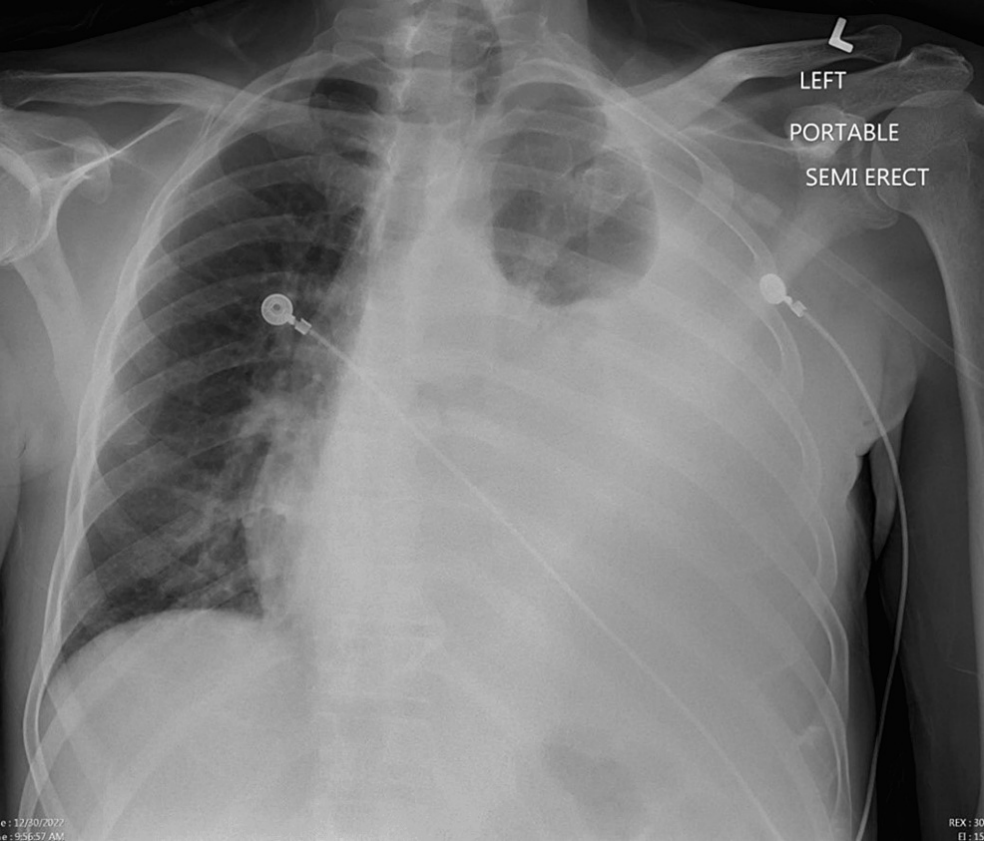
A chest X-ray demonstrating large volume left-sided pulmonary effusion with mass effect resulting in a midline shift of mediastinal structures and trachea.
